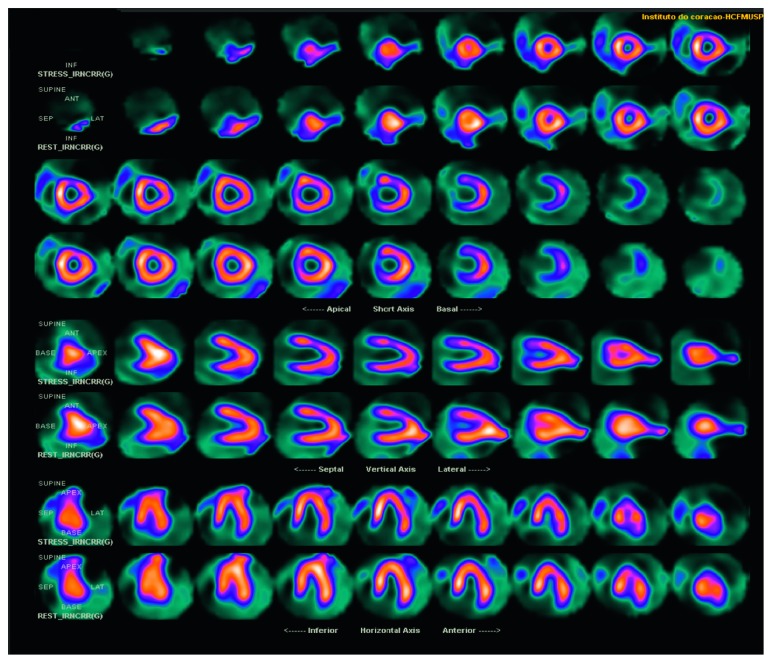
Sesta-MIBI perfusion scintigraphy showing discrete anterior wall perfusion defect (apical segment) and transient perfusion defect in the mild and basal segments of the anterior wall and in the middle segment of the anterolateral wall.
